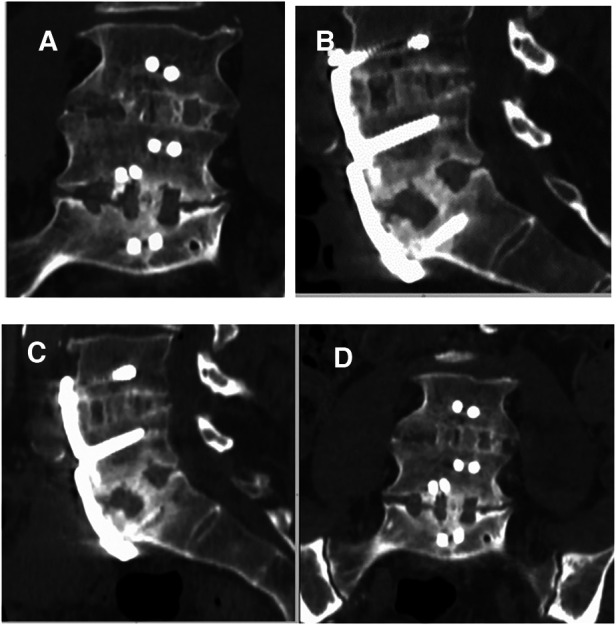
Two-level ALIF using BIOBank allograft on a 58-year-old male at L4L5 et L5S1. Coronal and sagittal computed tomography scans taken 14 months (A,B) and 36 months (C,D) after surgery showing satisfactory fusions.
